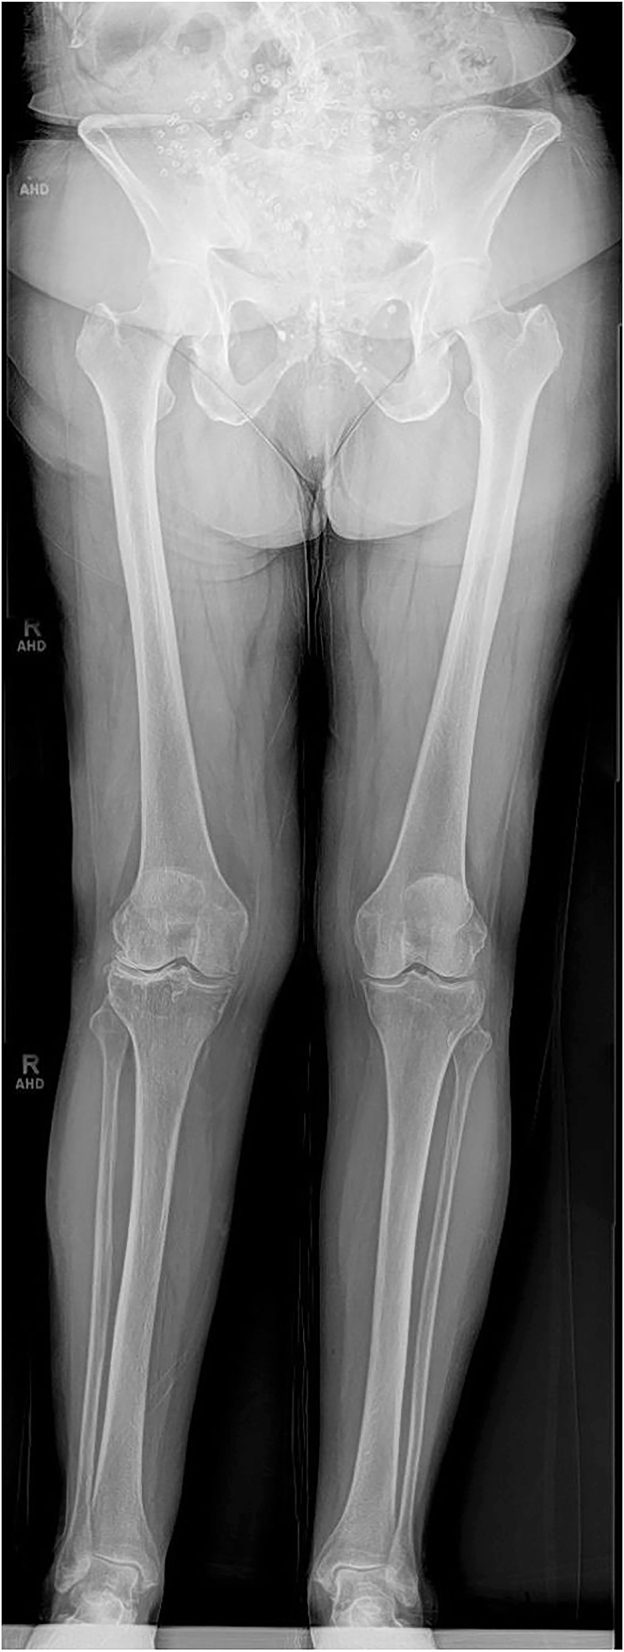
Coronal plane imaging. Full-length radiograph while the patient is bearing weight on the limb.
